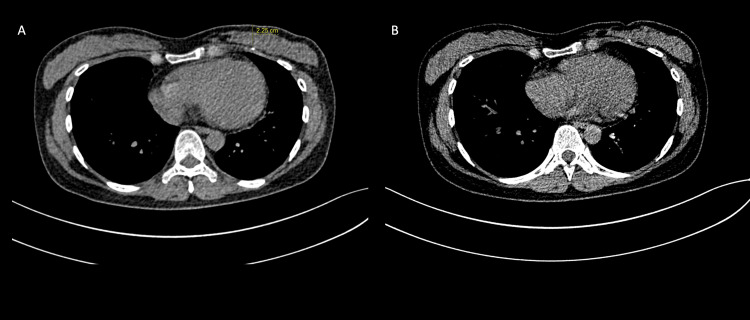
CT-guided fluoroscopy revealing the tumour marker positioned at a depth of 2.2 cm from the skin (A). An 'X' demarcation was meticulously placed on the cutaneous surface directly above the tumour marker, facilitating precise localisation for our surgical colleagues.
